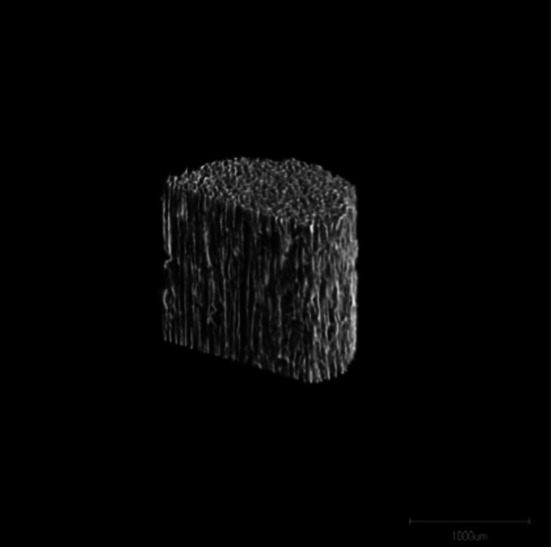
Three-dimensional micro-computed tomography image of the novel unidirectional porous hydroxyapatite (provided by Kuraray Co., Ltd.) showing unidirectional pores in the vertical direction and some interconnection toward the horizontal direction at the top
